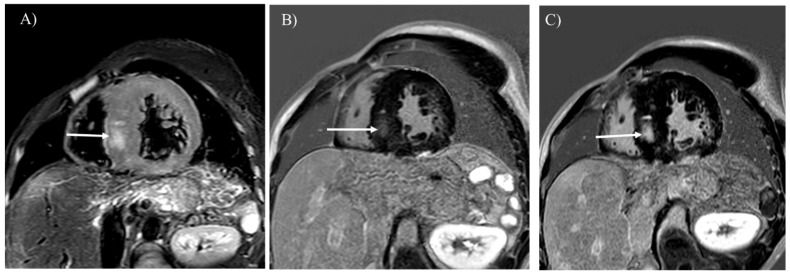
T2-weighted imaging (A) showed myocardial hypertrophy and edema in a 27-year-old man with glycogen storage disease type IIIa (arrow). He had undergone liver transplantation 8 years before. Late gadolinium enhancement (LGE) imaging (B) identifies a myocardial scar in the hypertrophied septum (arrow). The location of LGE is identical to that of myocardial edema (A,B); (arrows). Progression of the myocardial scarring was observed at 30 years (C), (arrow).
